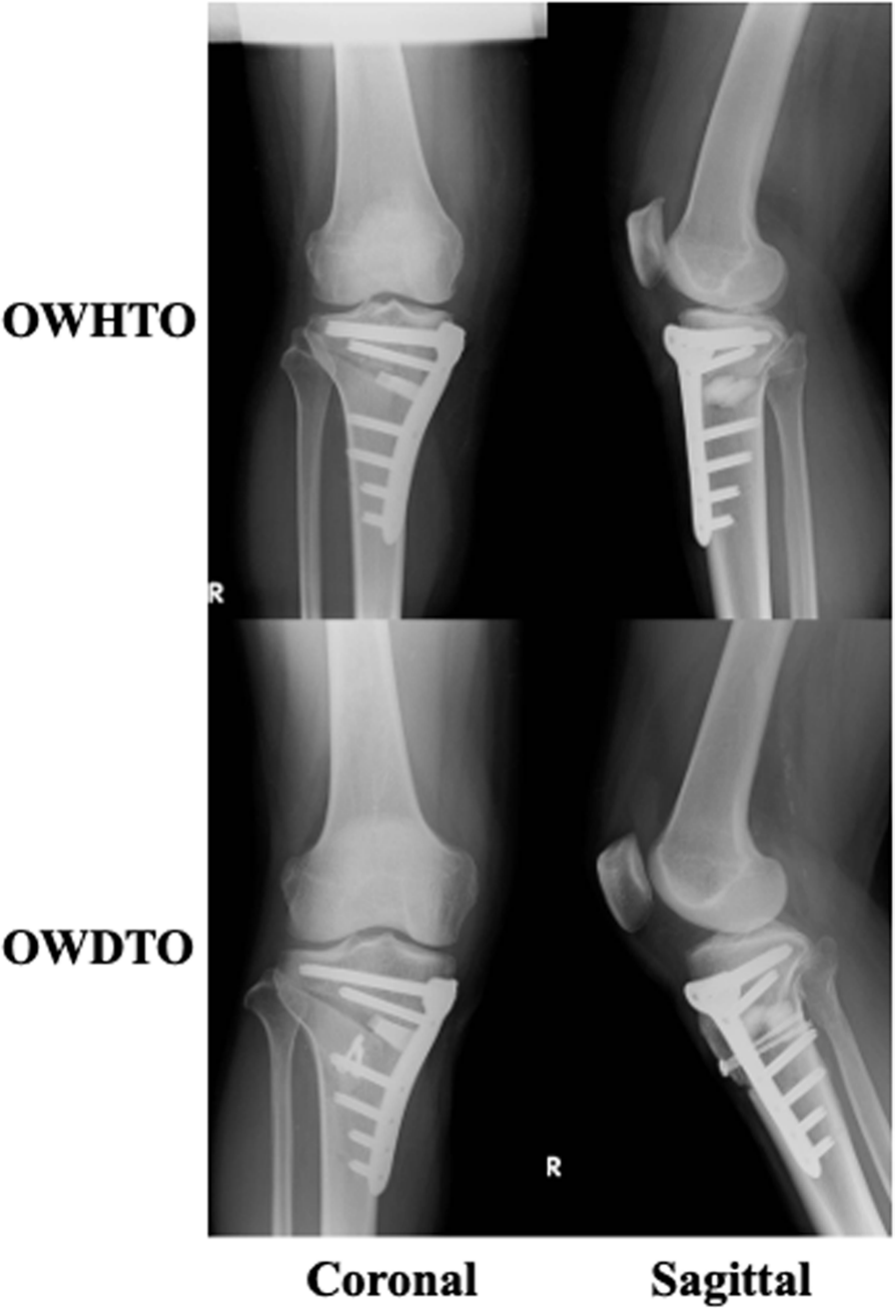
Representative X-ray of OWDTO and OWHTO
